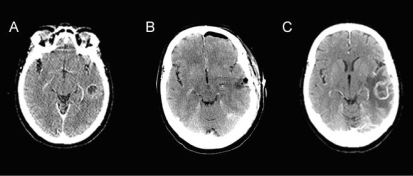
Post-contrast computed tomography (A) before resection demonstrates a part-solid, part-cystic, ring-enhancing lesion in the left temporal lobe; (B) immediately following resection demonstrates a resection cavity containing air and fluid without an enhancing abnormality (left frontal postsurgical pneumocephalus); (C) 2 months post-resection demonstrates expansion of ring enhancement within the left temporal lobe, associated with increased perilesion edema and mass effect.
